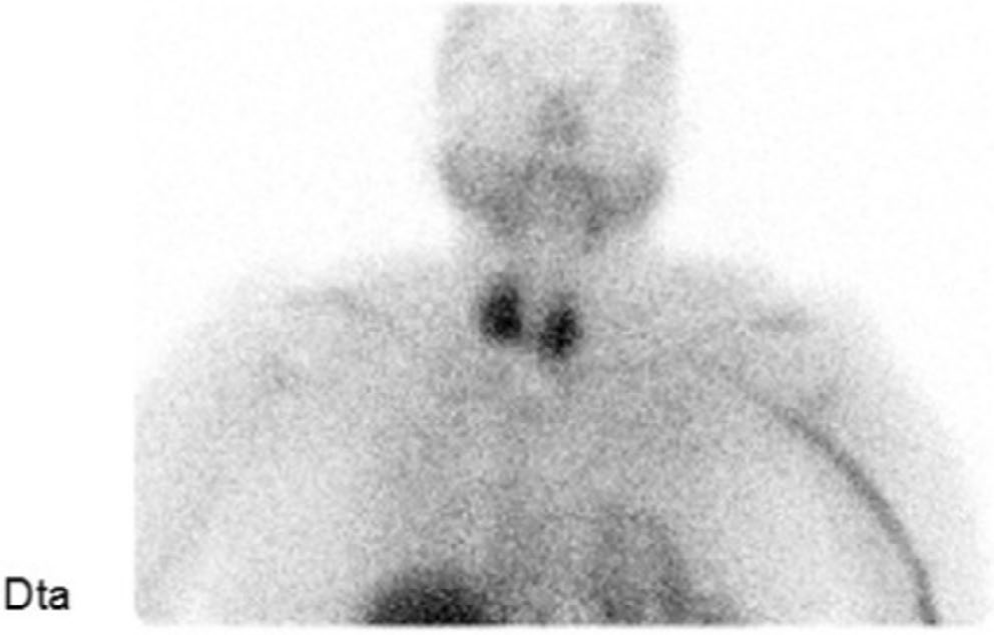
Repetition of ^99m^Tc‐pertechnetate thyroid scintigraphy (coronal view); diffuse increased uptake, compatible with type 1 AIT.
